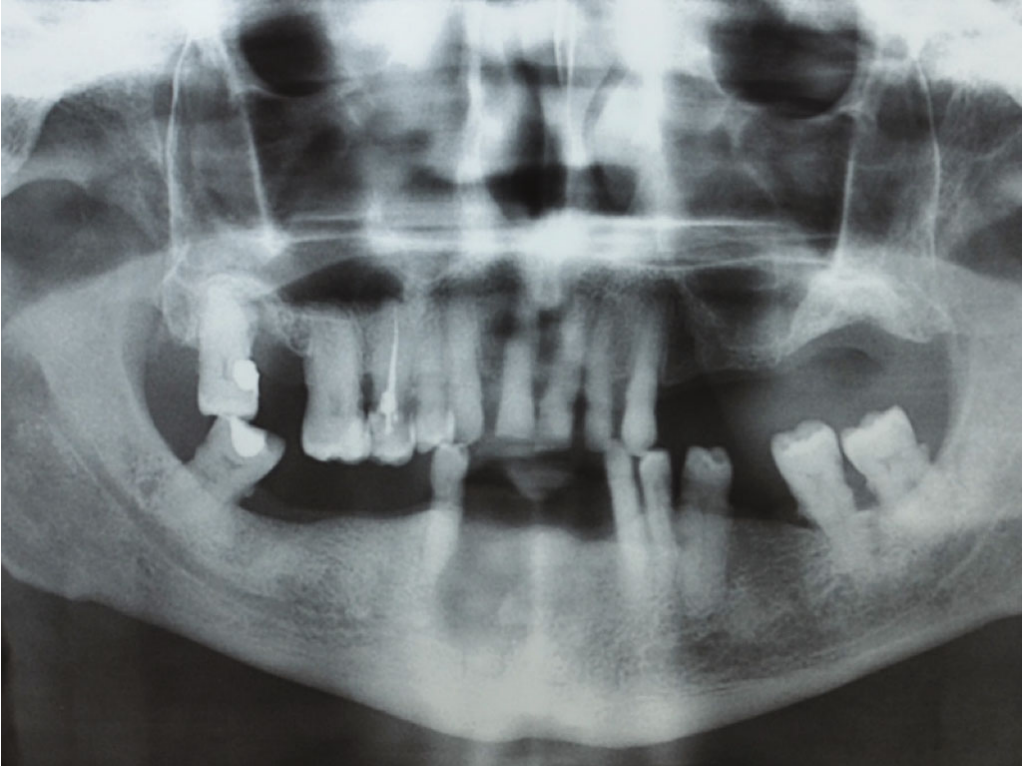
Radiographic signs of MRONJ.
